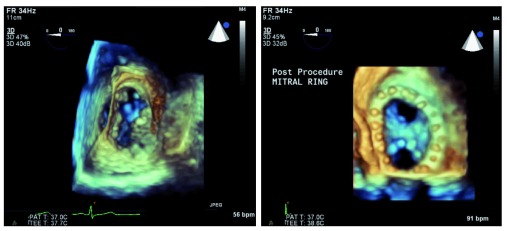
Three-dimensional assessment of the mitral valve has led to improved identification of pathology and guided repair. The native mitral valve (left) shows an anterior leaflet cleft and a posterior leaflet prolapse. Surgical repair includes mitral annuloplasty ring and Alfieri stitch (right), resulting in two distinct inflow orifices. 3D, three-dimensional; bpm, beats per minute; PAT T, patient temperature; TEE T, transesophageal echocardiography temperature.
